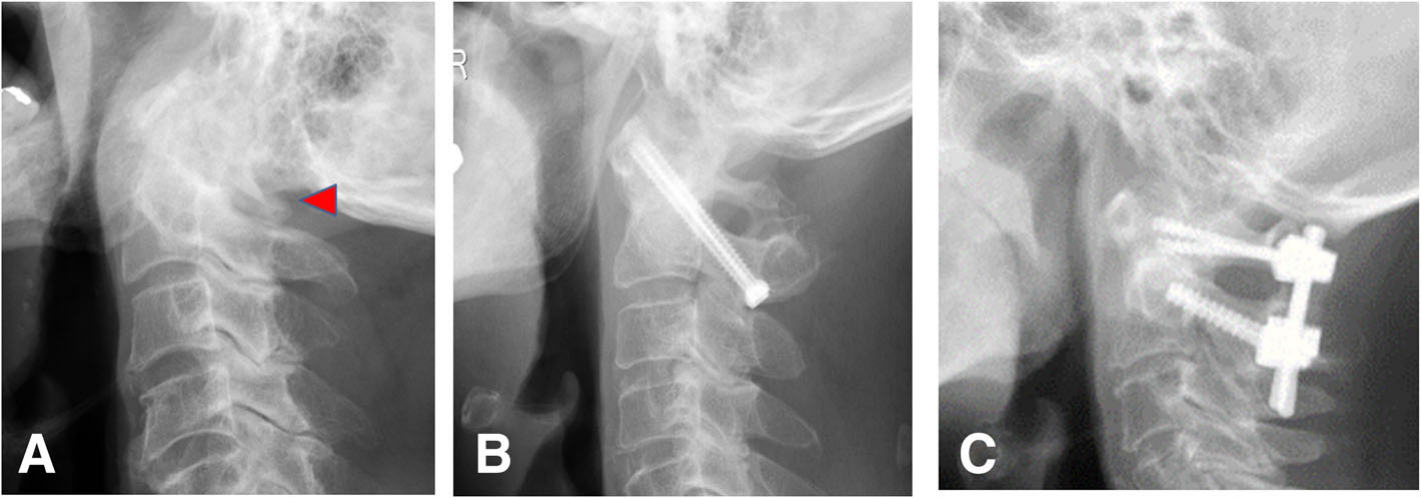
Representative lateral view of cervical spine X-rays post-operation. a C1 laminotomy. Posterior arch of C1 is decompressed (arrow head). b Transarticular screw fixation (Magerl and Brooks technique). c C1/2 fixation using C1 lateral mass and C2 pedicle screw
